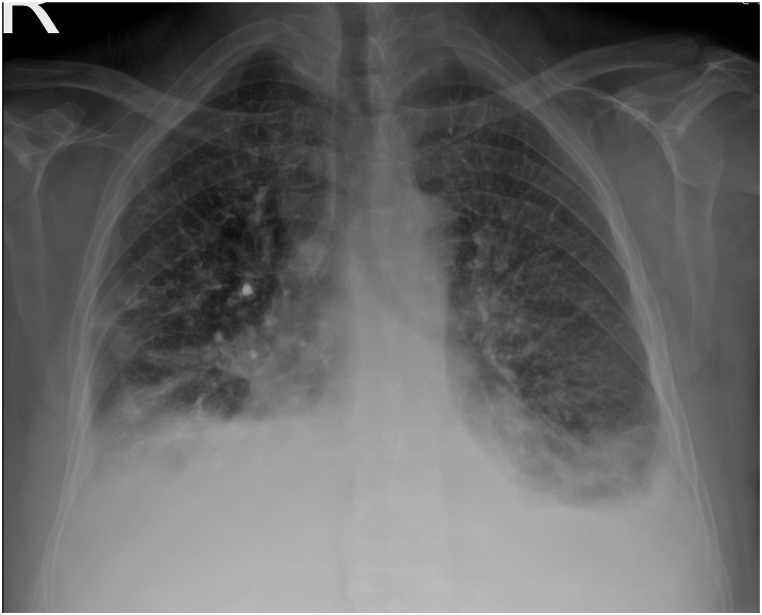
Chest radiograph on postoperative day 7 demonstrating mild reduction in the right pleural effusion and a mild increase on the left side, with bilateral pulmonary opacities.
